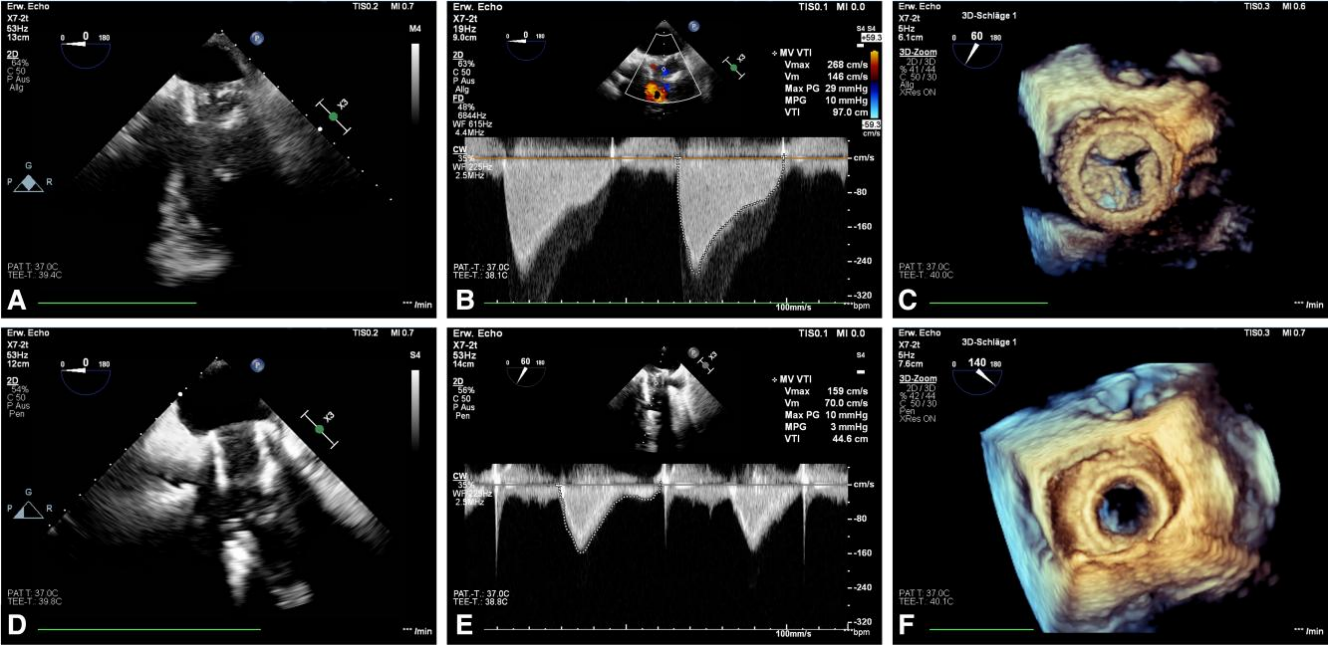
Echocardiographic findings depicting a degenerated bioprosthetic valve with a mean pressure gradient of 10 mmHg and a peak velocity of 268 cm/s without any visible paravalvular leakage. (*A–C*) Echocardiographic findings showing the full mobility of the newly implanted transcatheter mitral valve with complete leaflet motion and a major reduction of the mean pressure gradient to 3 mmHg and a peak velocity of 159 cm/s without any visible paravalvular leakage (*D–F*).
